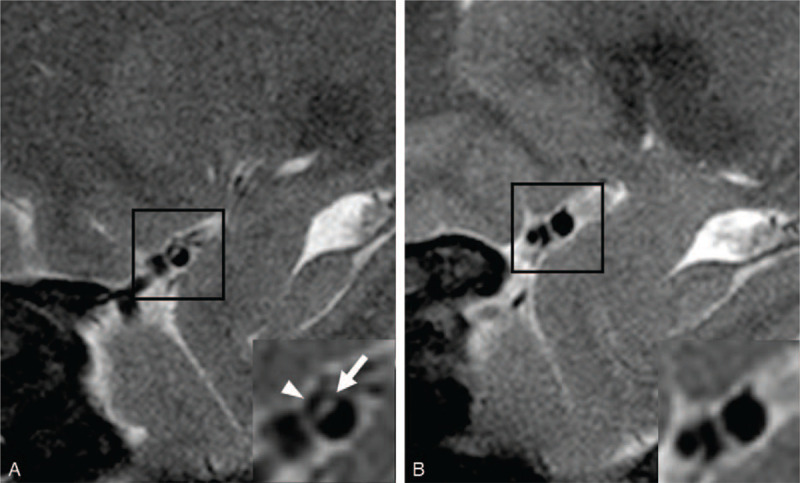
A, T2-weighted HR-MRI shows the intimal flap in the right MCA (arrow). Note the dorsally-located pseudo-lumen with absence of intramural hemorrhage (arrowhead). B, T2-weighted HR-MRI at three-month revisit shows resolution of the MCA dissection lesion. HR-MRI = high-resolution magnetic resonance imaging, MCA = middle cerebral artery.
